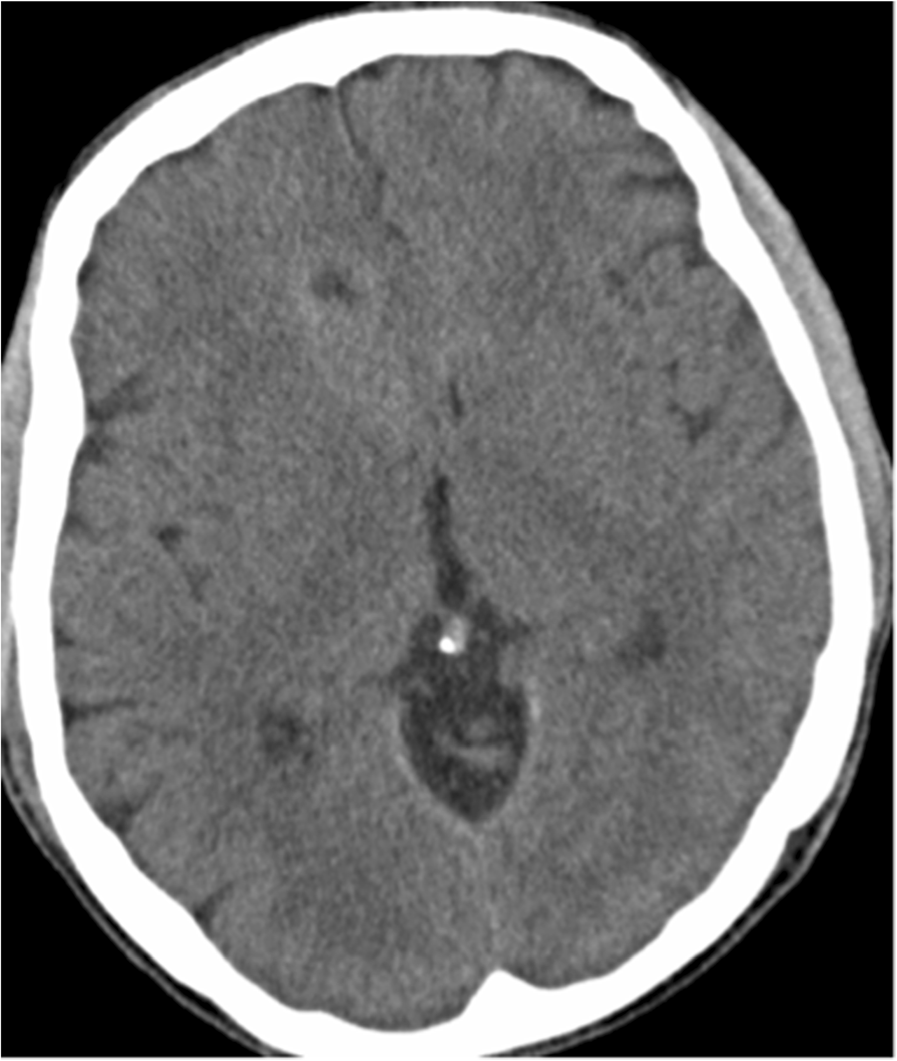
Preoperative non-contrast axial computed tomography showing a hypodense lesion with peripheral bleeding in the right anterior cingulate gyrus.
